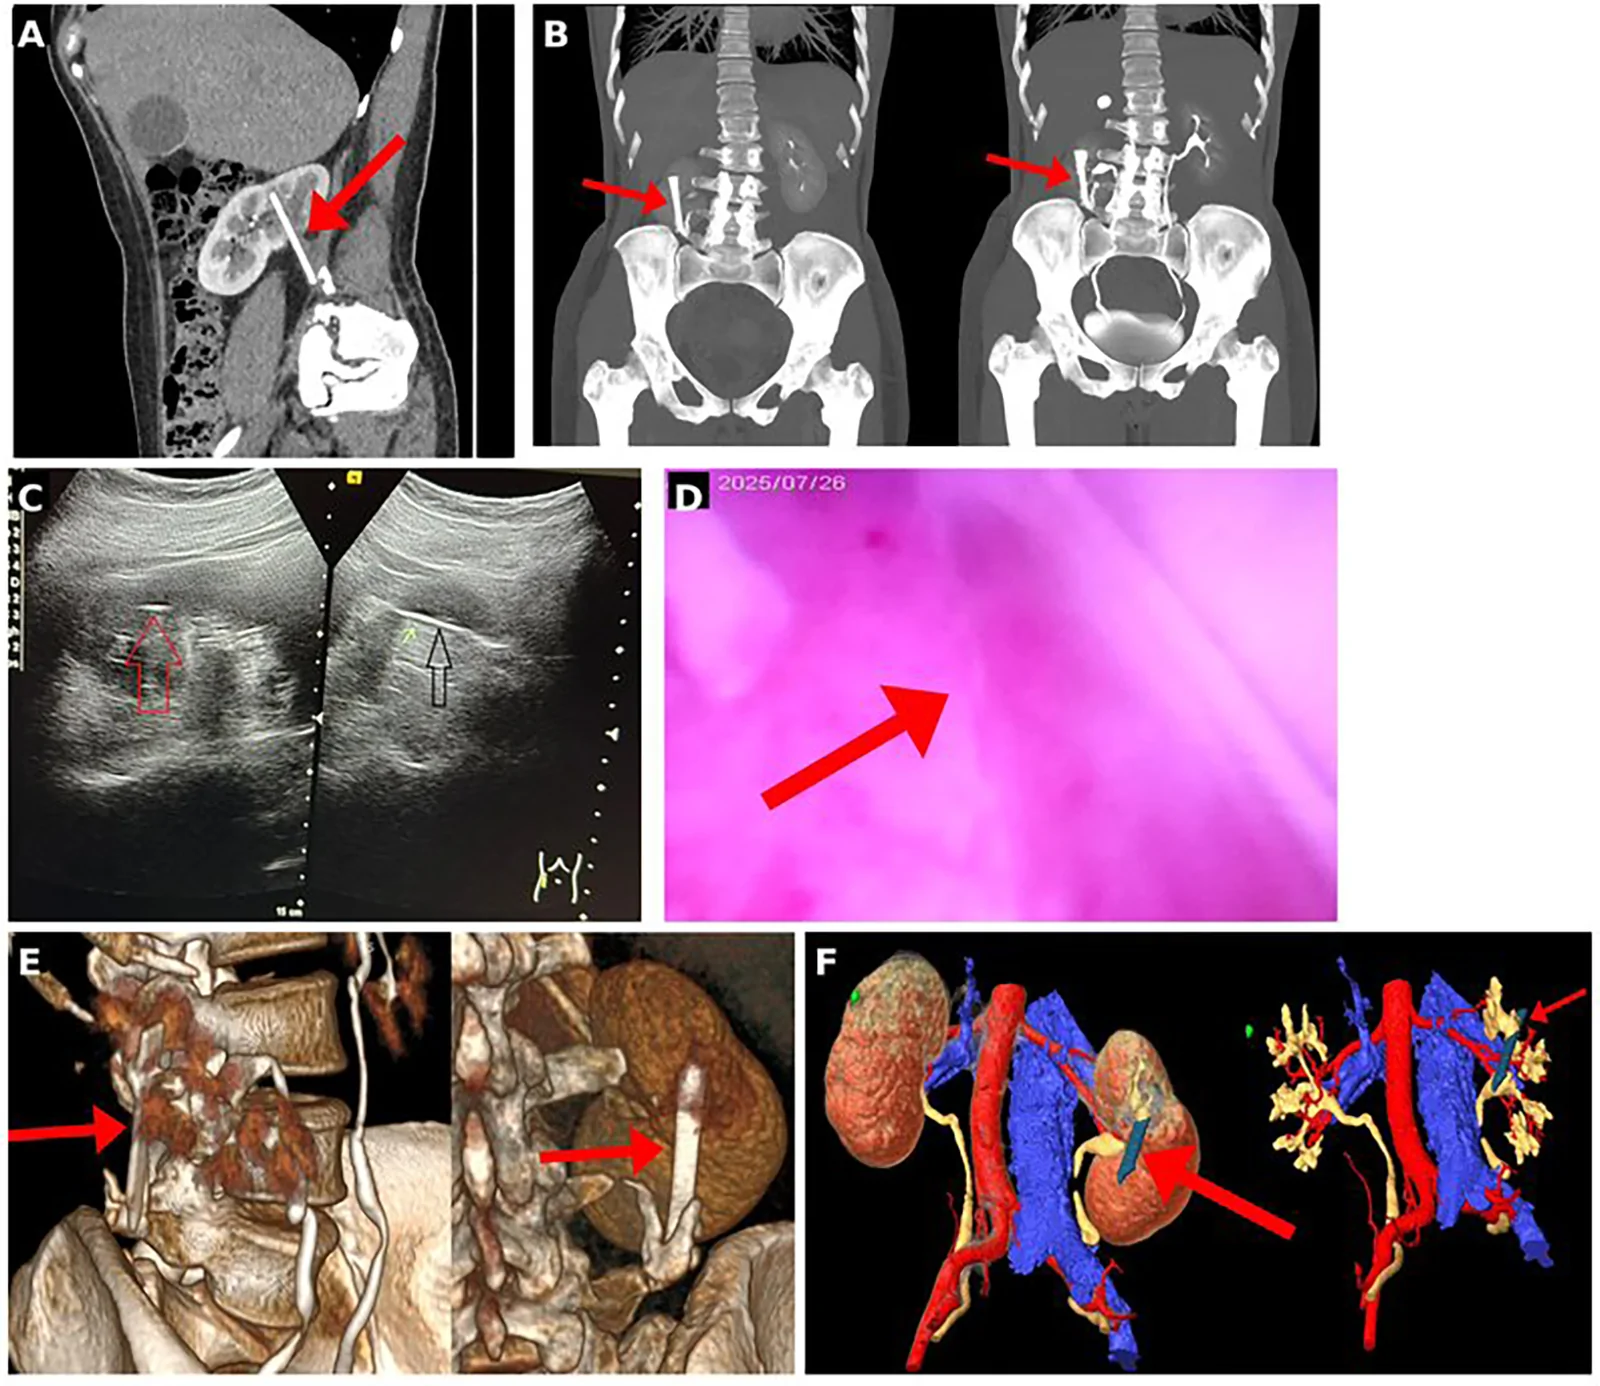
Preoperative multimodality imaging. (A) CE-CT sagittal reconstruction: hyperdense glass foreign-body material (arrow; ∼1,150–1,200 HU) extending from the right L5 transverse process through quadratus lumborum into the upper renal pole; the foreign body was present as two fragments along this track. (B) CE-CT coronal reconstruction, excretory phase: the foreign body (arrows) traverses renal parenchyma and enters the upper calyces, with no hydronephrosis or contrast extravasation; the proximal (quadratus lumborum) and distal (renal parenchyma) fragments correspond to the two components removed at surgery. (C) Transabdominal ultrasound, longitudinal view: linear hyperechoic foreign-body material (arrow) projecting beyond the renal capsule; colour Doppler confirms preserved segmental perfusion. (D) Flexible ureterorenoscopy: direct endoscopic visualisation of glass (arrow) within the renal pelvis. (E,F) Volumetric 3D CT reconstructions (posterior-oblique and anterior views): colour-coded segmentation shows the foreign body (teal) relative to the right kidney (orange), aorta (red), IVC (blue), and vertebral column (beige), delineating the oblique trajectory and supporting the absence of major vascular encasement.
